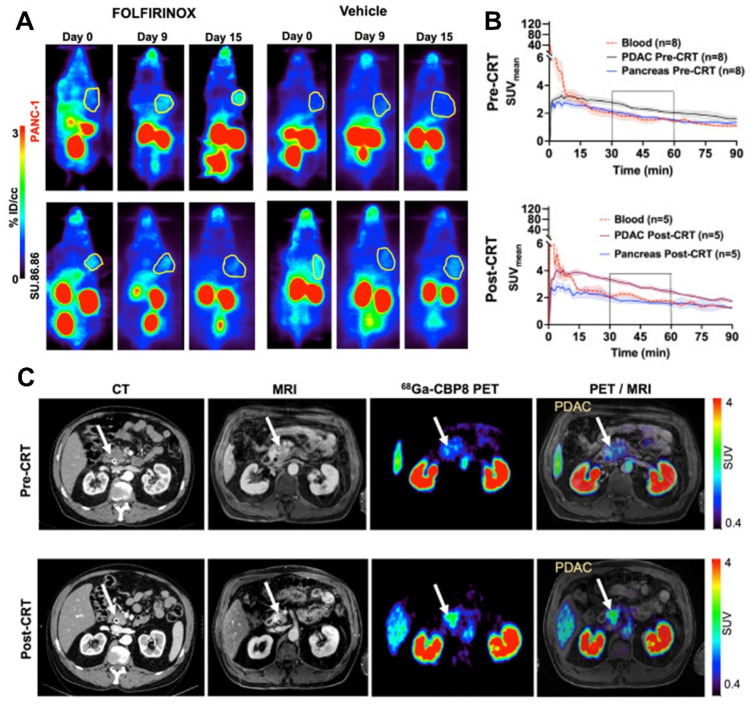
^68^Ga-CBP8 PET captures treatment-induced collagen remodeling in PDAC models and patients. (A) Quantitative tumor uptake of ^68^Ga-CBP8 at days 0, 9, and 15 following FOLFIRINOX or vehicle treatment. Tumors are shown in yellow circle. (B) Dynamic PET time–activity curves (0–90 min) showing rapid blood clearance, with blood SUVmean approaching uninvolved pancreas SUVmean by 15–30 min. (C) Representative axial images before and after CRT: contrast-enhanced CT (late arterial phase), gadolinium-enhanced MRI (portal venous phase), ^68^Ga-CBP8 PET (30–45 min), and fused PET/T1-weighted MR. PDAC tumor is shown with a white arrow. *CRT*, chemoradiotherapy. Reproduced from ref. [18]. Available under a CC-BY 4.0 license.
